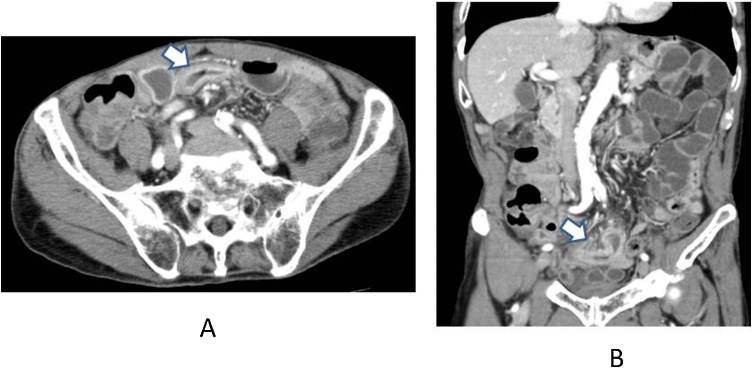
CT on 23th day after onset of HPVG. CT revealed thickness of intestinal wall which was a same portion of intestinal pneumatosis and fluid collection in oral intestine (Fig. 3A and 3B: indicated by white arrow).
